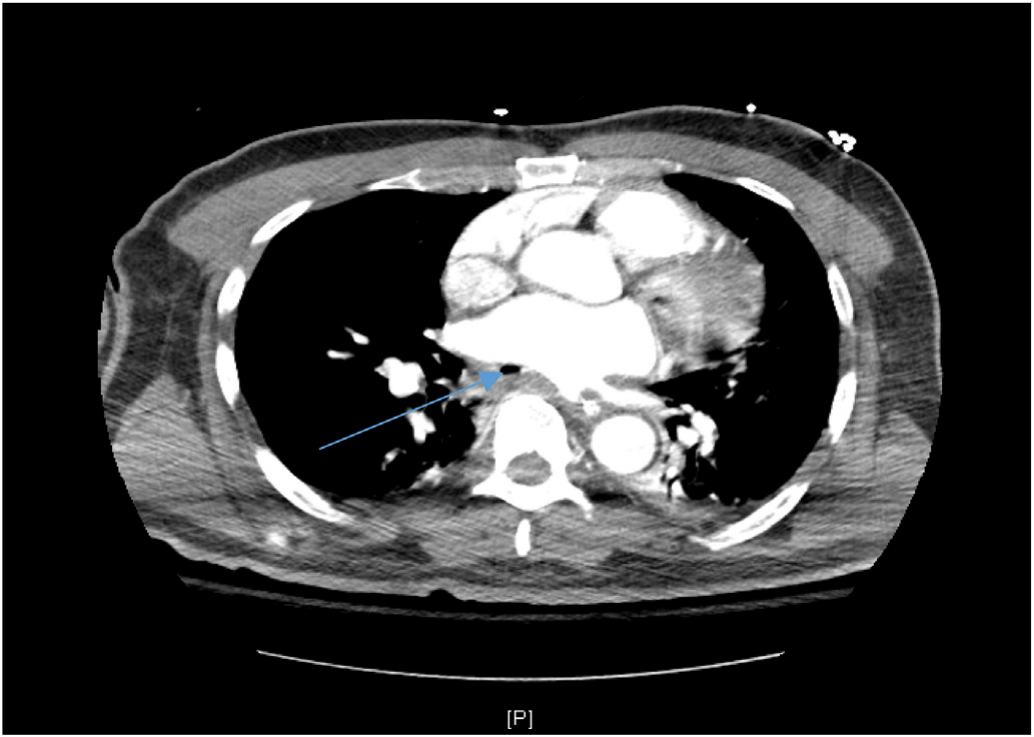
CTA chest with IV contrast demonstrating an outpouching off the posterior wall of the left atrium at the level of the inferior pulmonary vein, consistent with an atrioesophageal fistula (blue arrow). (For interpretation of the references to color in this figure legend, the reader is referred to the Web version of this article.)
